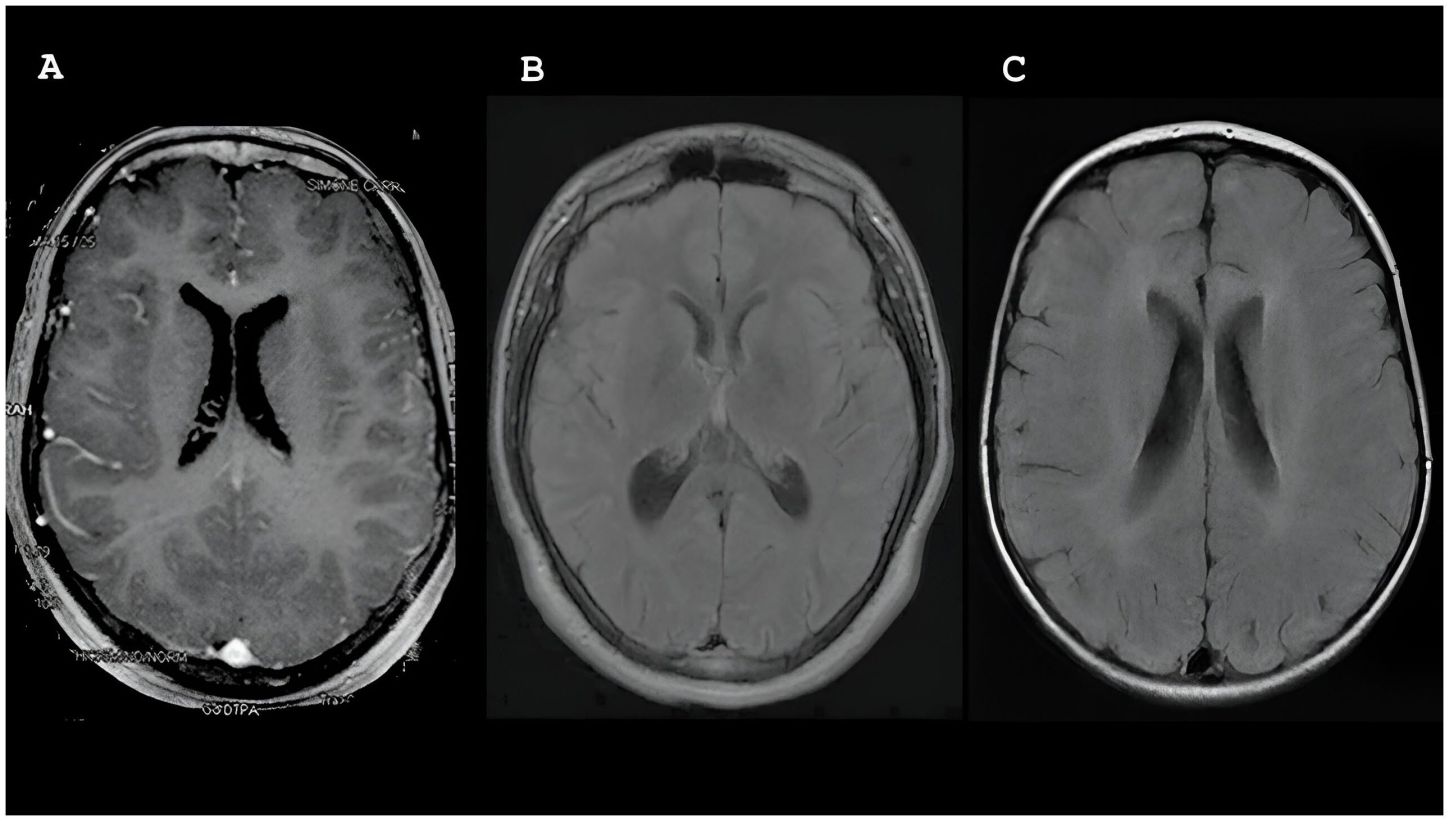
Magnetic resonance imaging (MRI) showing white matter changes in participants P4, P5 and P7.
